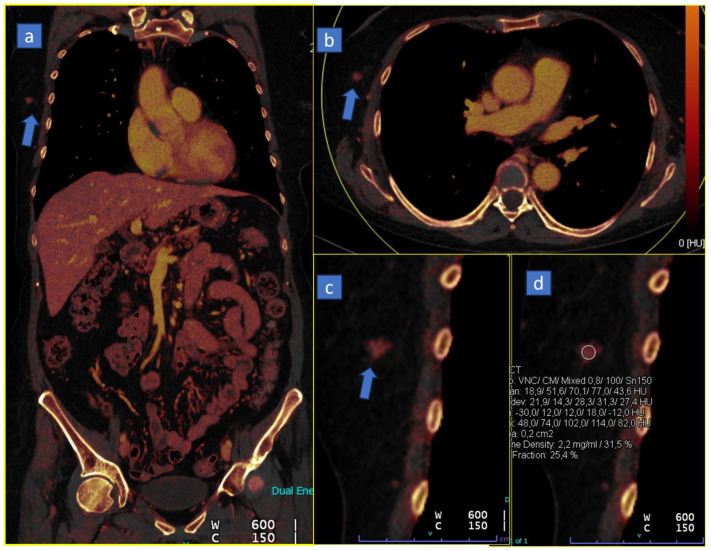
Seventy-one-year-old woman suffering from rectal cancer with incidental breast cancer on a DECT scan. On the coronal DECT scan acquired during venous phase, (a) a tiny hypervascular breast nodule is recognized (arrow). On the corresponding axial scan, (b) the lesion shows irregular margins (arrow). On the coronal CT cropped image, (c) the nodule shows clearly spiculated margins (arrow). By positioning an ROI (d), the assessment of DECT numbers calculated from an iodine map shows a clear enhancement of the solid tumor (ROI).
